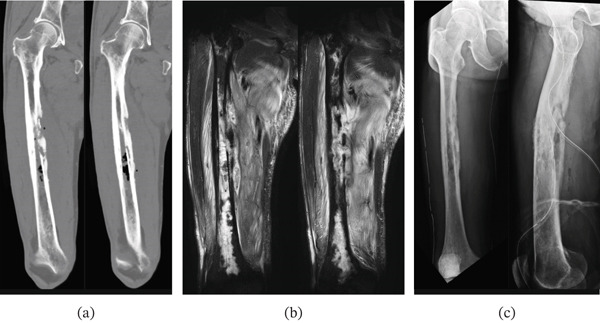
Case 2. (a) Preoperative coronal CT image of the right hip and femur at readmission, (b) coronal MRI with STIR image of the right hip and femur at readmission, and (c) postoperative anteroposterior and lateral radiographs of the right hip and femur.
